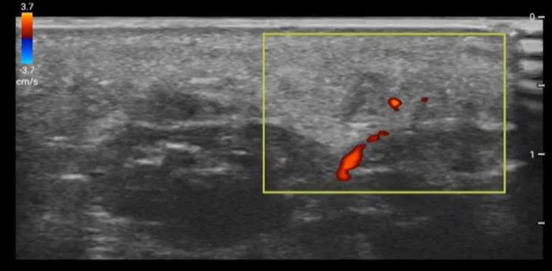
Color Doppler was applied near a second anechoic track (arrows). Lack of color flow confirmed anechoic lesion was not a vascular structure.
